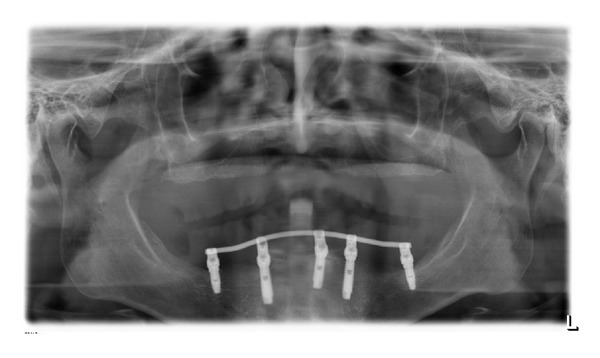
Panoramic X-ray after one-year follow-up.
